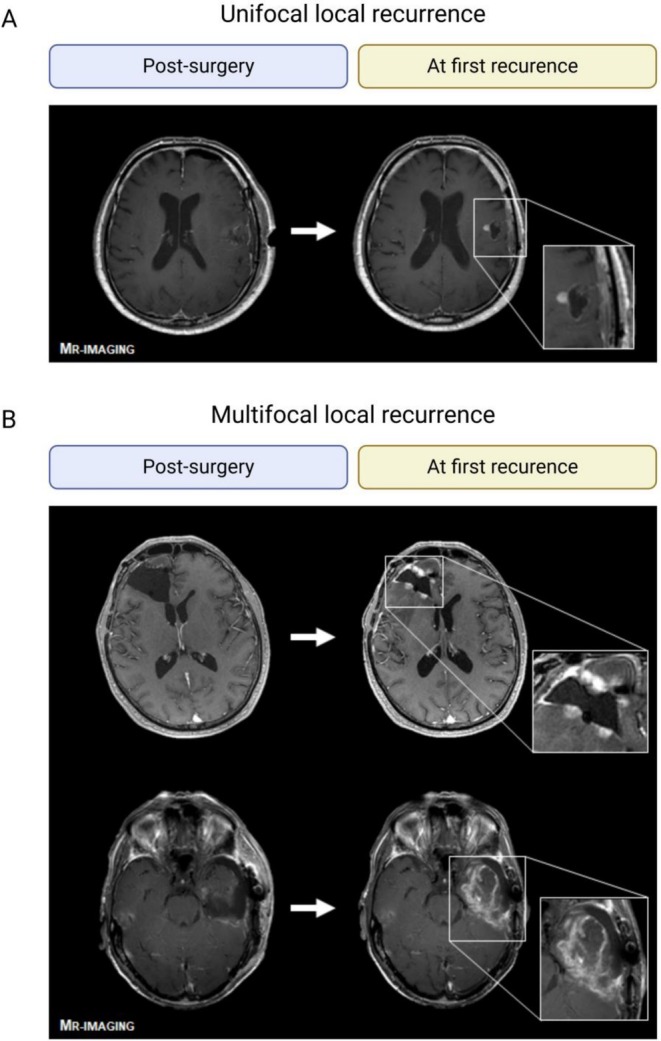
Recurrence patterns based on MR‐Imaging. Comparison of MR‐imaging post‐surgery and at first recurrence with examples of local unifocal recurrence with a single contrast‐enhanced lesion (A) or local multifocal recurrence with > 1 CE‐lesion (B, upper MRI) or even affection of the entire margins (B, lower MRI). Created in BioRender. Feldheim, J. (2023) BioRender.com/p08j063.
